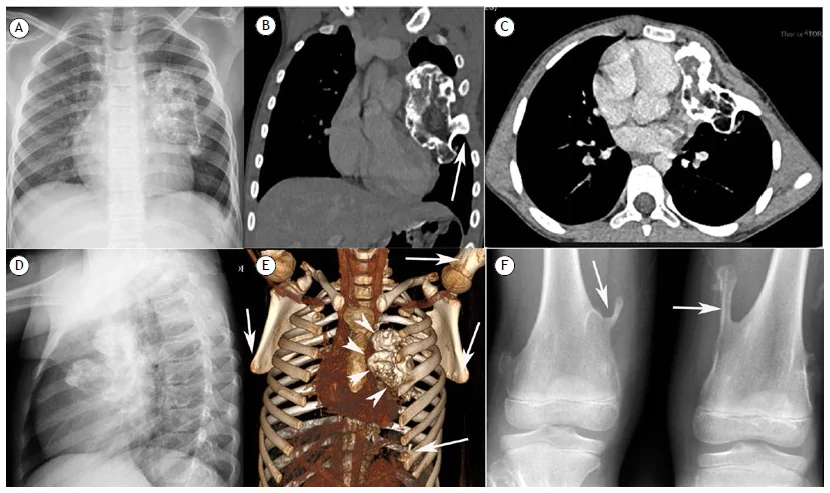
Frontal (A) and lateral (B) chest radiographs showing an irregular calcified mass in the left hemithorax. (C) Coronal reconstruction with mediastinal window setting demonstrated that the partially calcified mass originated mainly from the left fourth costal arch (arrow). (D) Three-dimensional coronally reconstructed image showing that the lesion was related to the third and fourth ribs (arrowheads). Also note exostoses in the scapulae, left humerus, and left seventh rib (arrows). (E) Axial section showing the relationship of the mass to mediastinal structures. (F) Radiograph of the knees showing pedunculated osteochondroma in both femurs (arrows).
